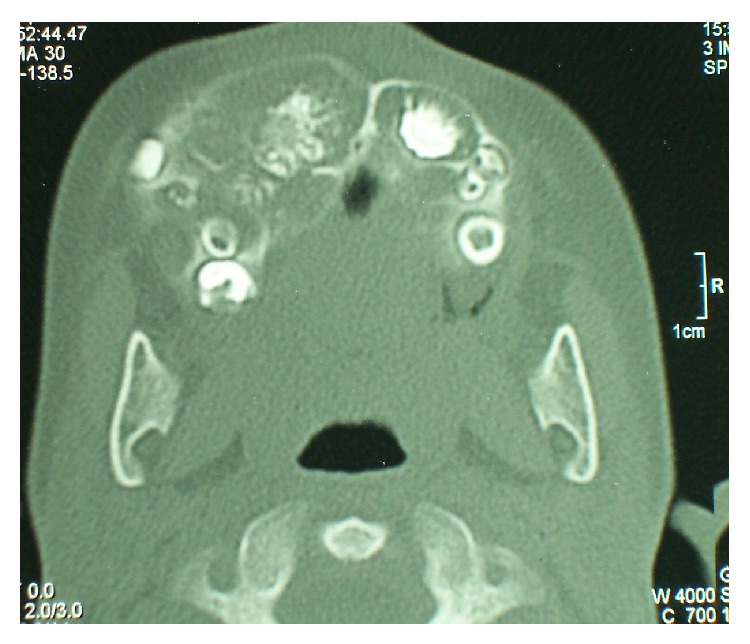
CT scan revealing hyperdense mass involving the labial cortex and pterygoid plates.
